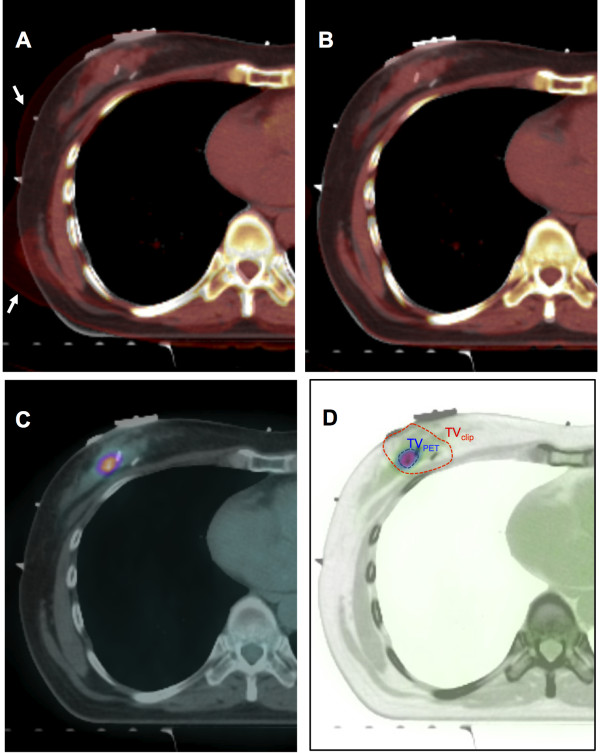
Tumor bed localization procedure using deformable image registration of diagnostic PET-CT. A. Automatic rigid image registration (DIR) process (arrows indicate the CT component of PET-CT), B. Deformable image registration (DIR) process between planning CT and CT component of PET-CT, C. Overlapped image between planning CT and PET component from PET-CT after DIR process, D. Target volume delineation of TV_PET_ and TV_clip._
